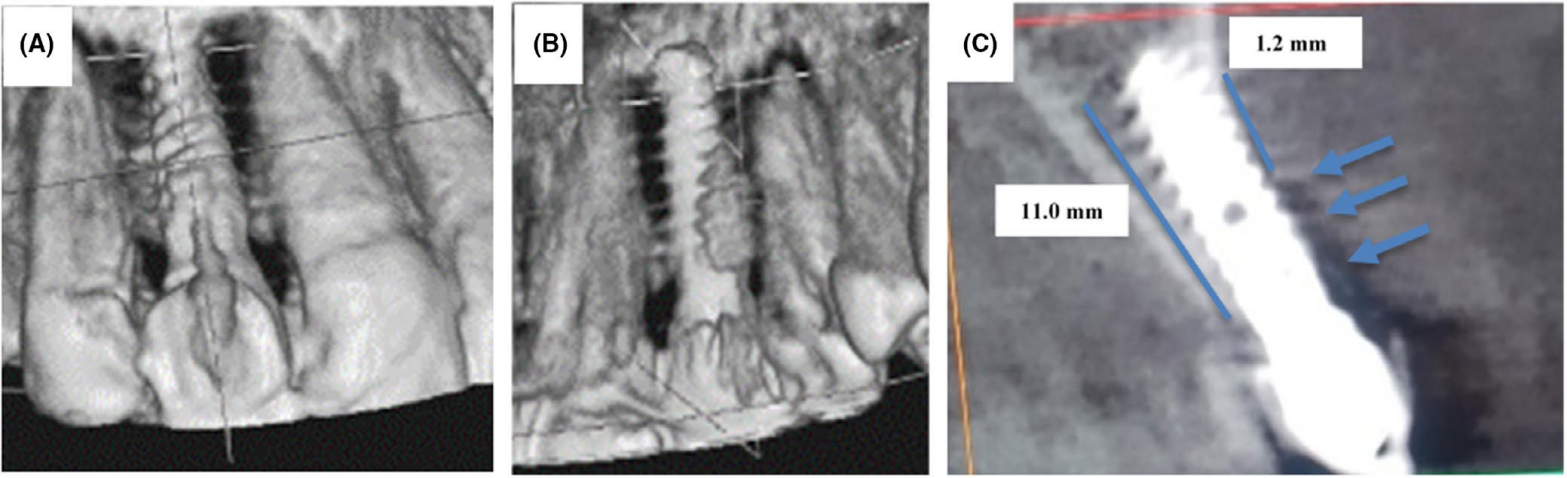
3D composition of the implant site before treatment in labial view (A) and palatal view (B). (C) The CBCT imaging of impant site showing the measurements of bone height in labioplatal view before treatment
